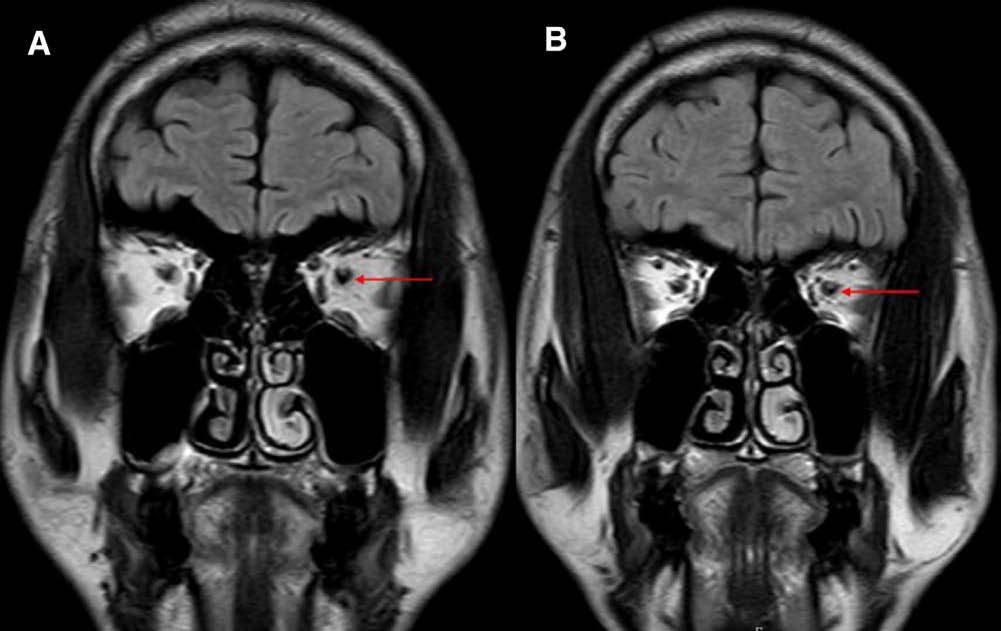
The MRI map during hospitalization. The dilatation of the optic nerve sheaths on coronal FLAIR images (red arrows). FLAIR = fluid-attenuated inversion recovery, MRI = magnetic resonance imaging.
